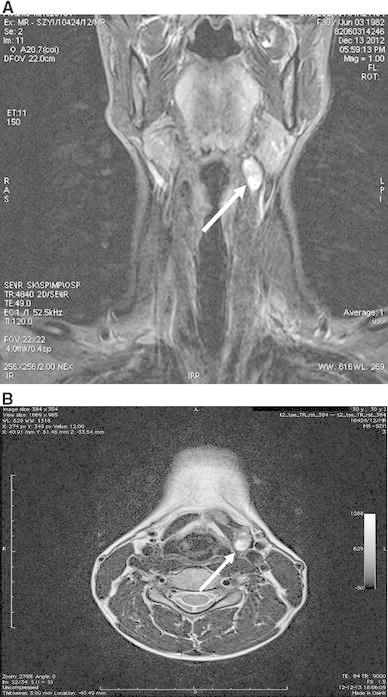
a T2-weighed MRI STIR (short inversion time inversion recovery) image in coronal plane. An *arrow* indicates an ectopic thyroid, b T2-weighed MRI STIR image in transverse plane. An *arrow* indicates a focal lesion in an ectopic thyroid
